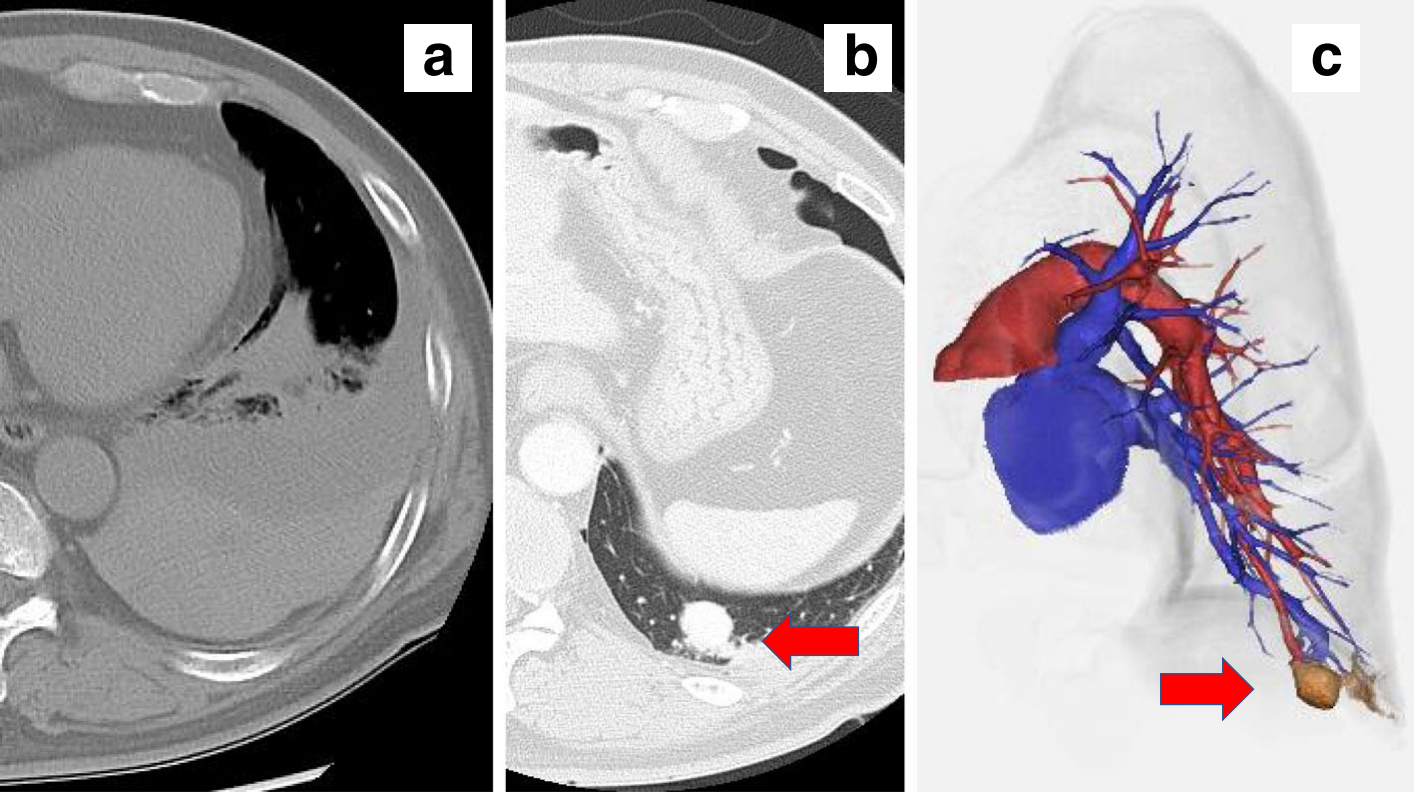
Chest computed tomography findings. a Chest CT showing left-sided hemothorax. b Chest CT after drainage showing a solitary nodule in the left lower lobe. c Three-dimensional CT showing the nodule to be suggestive of a PAVM
